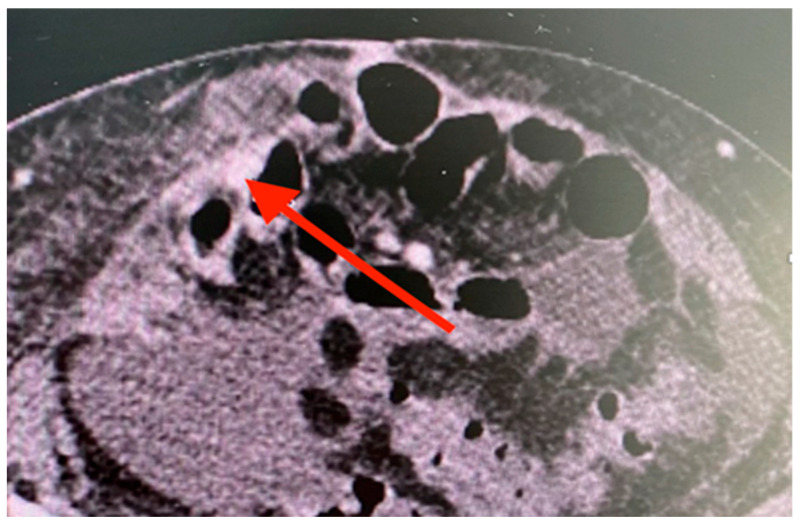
Ileal portocaval shunts. CT angiography image showing portocaval shunt in the ileum (red arrow); no active bleeding site was detected.
